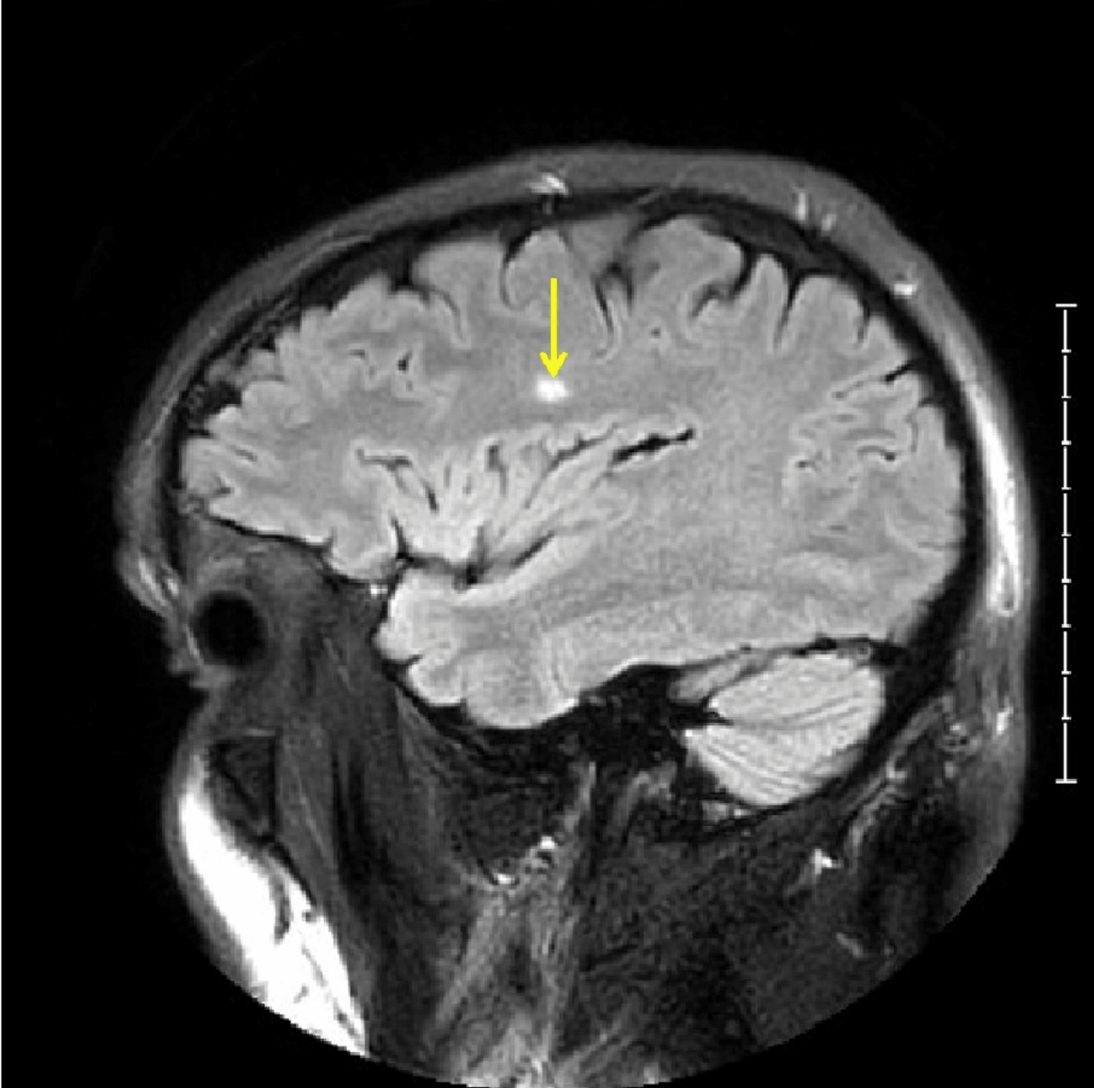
Brain MRI demonstrating a small focus of non-specific white matter hyperintensity in a patient with hemiplegic migraine. Sagittal FLAIR sequence showing a 7 mm hyperintense lesion within the deep white matter of the right frontal lobe (arrow). There is no associated diffusion restriction or mass effect. Such non-specific findings may represent sequelae of migraine-related vasogenic changes or chronic small-vessel ischemic alterations (Kellam score: 14.) FLAIR: Fluid-Attenuated Inversion Recovery
